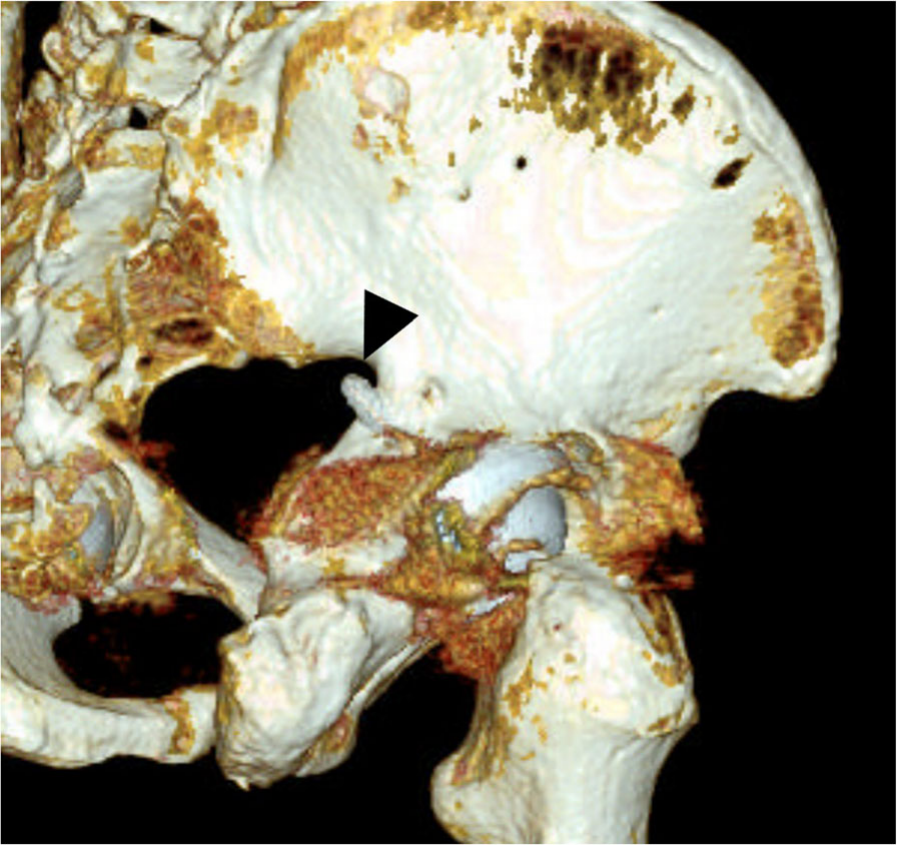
Oblique 3-dimensional computed tomography reconstruction showing an acetabular screw protruded into sciatic notch (arrowhead)
